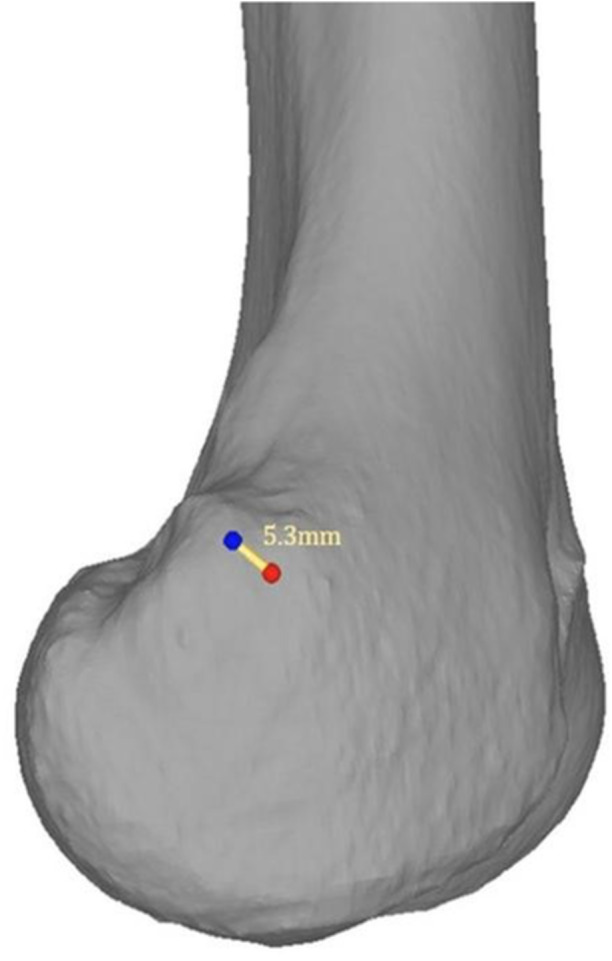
Distance between the radiographic (red) and anatomic (blue) Schoettle's point (SP) visualised in yellow. In this case, the distance measured 5.3 mm.
